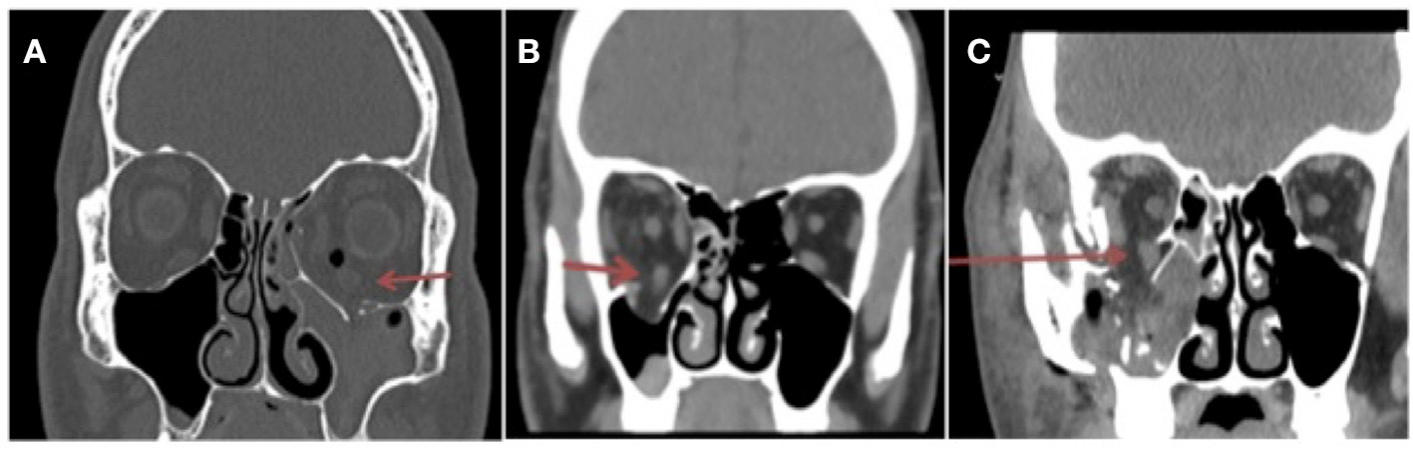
Coronal slice of facial bones CT demonstrating left orbital floor and medial wall fractures. Inferior rectus muscle is positioned (A) above the fracture plane (red arrow). The left maxillary sinus is obliterated and filled with blood. (B) The inferior rectus muscle is lying through the imaginary fracture plane. (C) The inferior rectus muscle falls below the fracture plane (red arrow) into the maxillary sinus.
